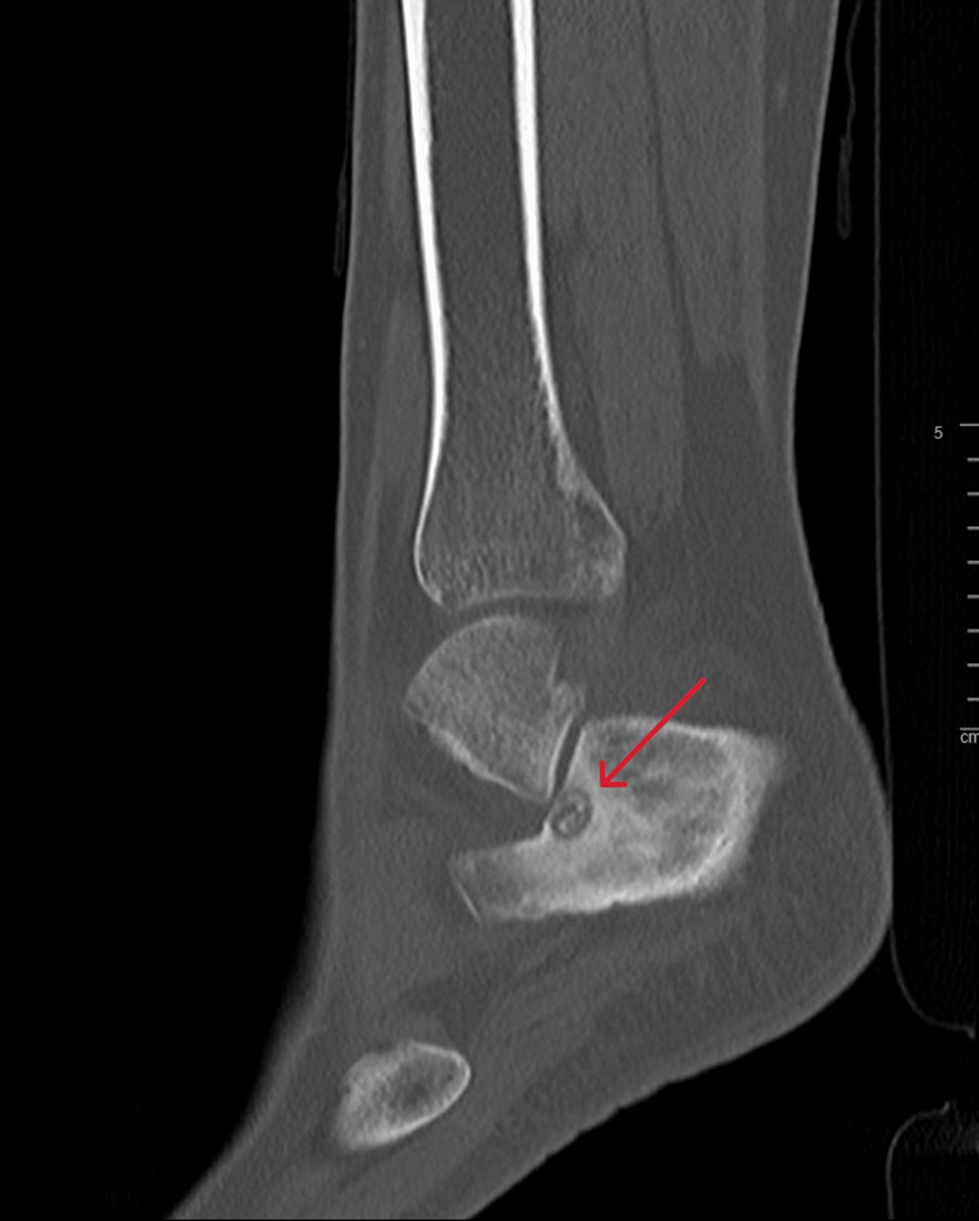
Sagittal view CT scan of the right ankle at the time of presentation CT: computed tomography
